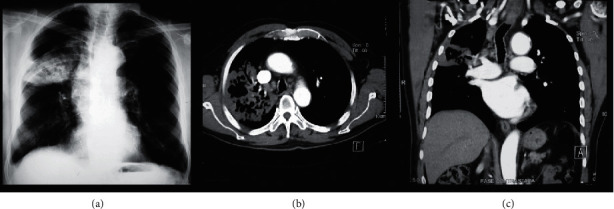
Necrotizing pneumonia due to *Histoplasma capsulatum*. (a) Chest X-ray (PA), with the presence of heterogeneous consolidation with radio lucid areas inside. (b, c) Chest CT showing lobar consolidation, cavitation in the right upper lobe, and parahilar lymphadenopathy.
